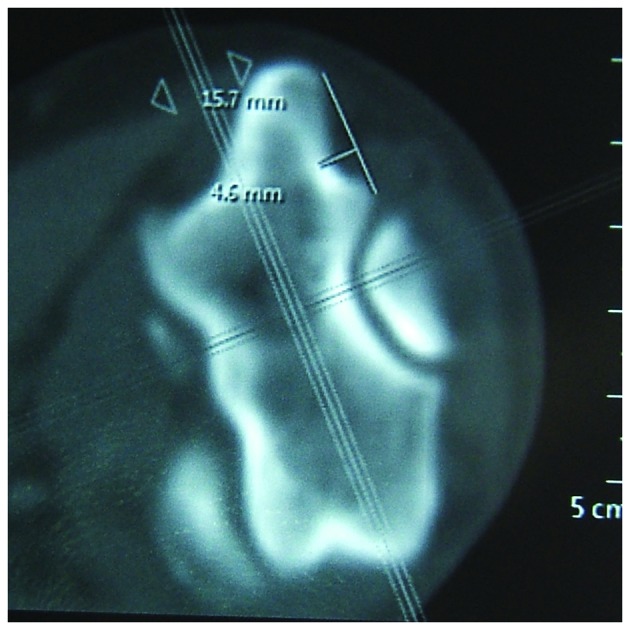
Normal adult cubital tunnel at 30° for a flat CT scan. CT, computed tomography.
